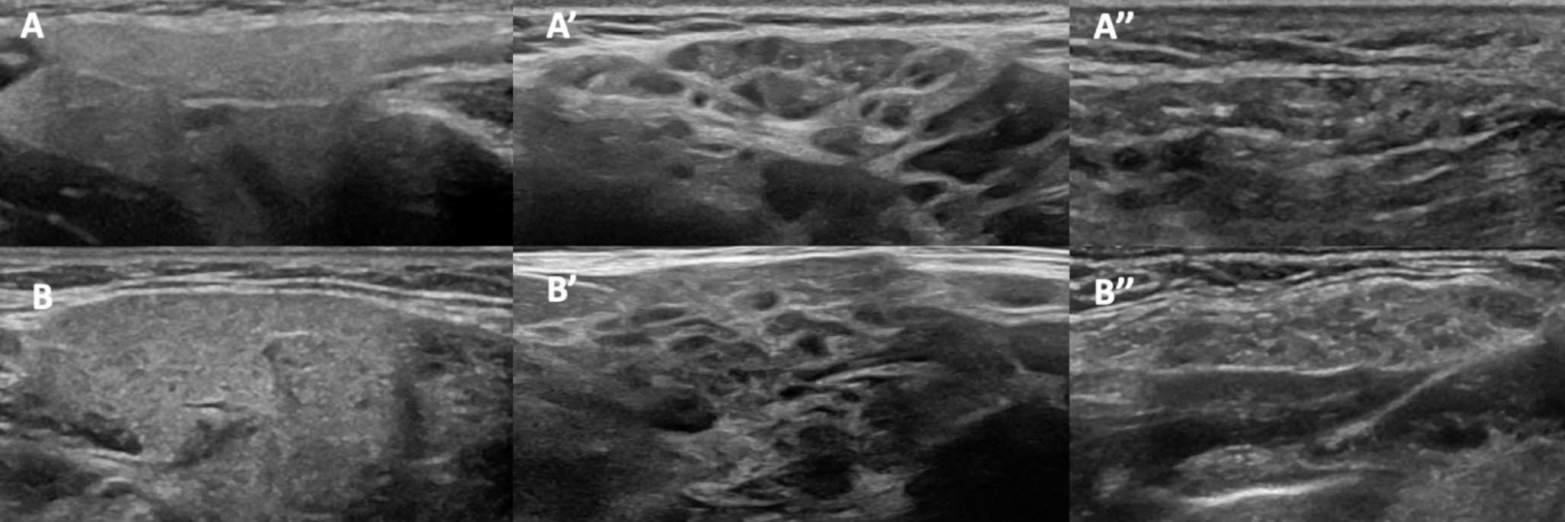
Ultrasound in the assessment of salivary glands. A and B present ultrasonographic images of the parotid and submandibular glands, respectively, showing the homogeneous structure of gland parenchyma in a healthy subject. A’ and B’ depict grade 3 inhomogeneity in patients with Sjögren disease, characterized by anechoic and hypoechoic areas affecting the entire gland surface (parotid and submandibular gland, respectively). A’’ and B’’ show diffuse atrophy and a loss of clear demarcation between the gland border and surrounding tissue in other patients with Sjögren disease (parotid and submandibular gland, respectively)
